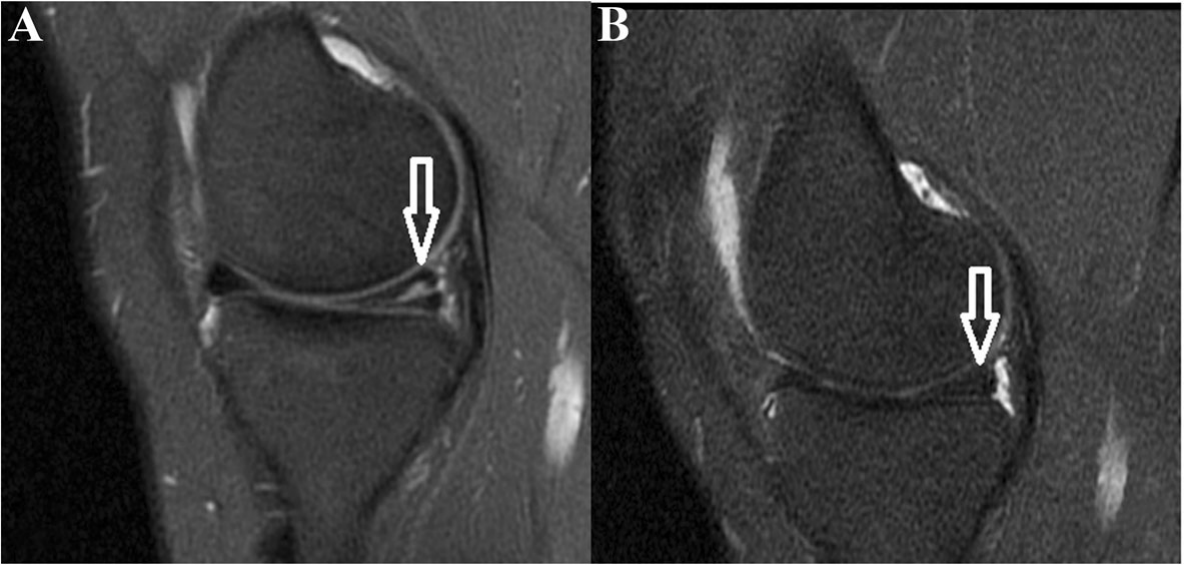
A sagittal T2-weighted MRI shows horizontal tear (*white arrow*) at the posterior horn of medial meniscus preoperatively (a) and postoperatively (b) who did not have a history of distinct previous trauma
